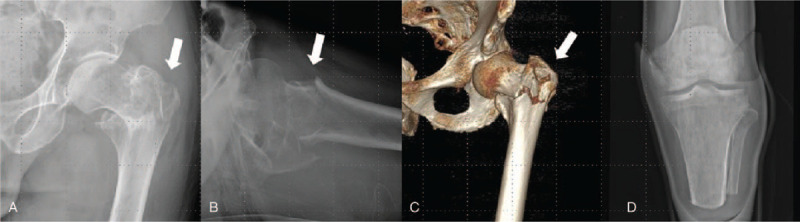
Case 1. (A) Plain radiographs of an 80-year-old man who fell and sustained an unstable left intertrochanteric hip fracture (white arrow, AO 31A3.3). (B) and (C) True lateral radiographs and 3-dimensional computed tomography image showing an unstable left intertrochanteric hip fracture. (D) Anteroposterior radiographs showing the below-knee amputation.
